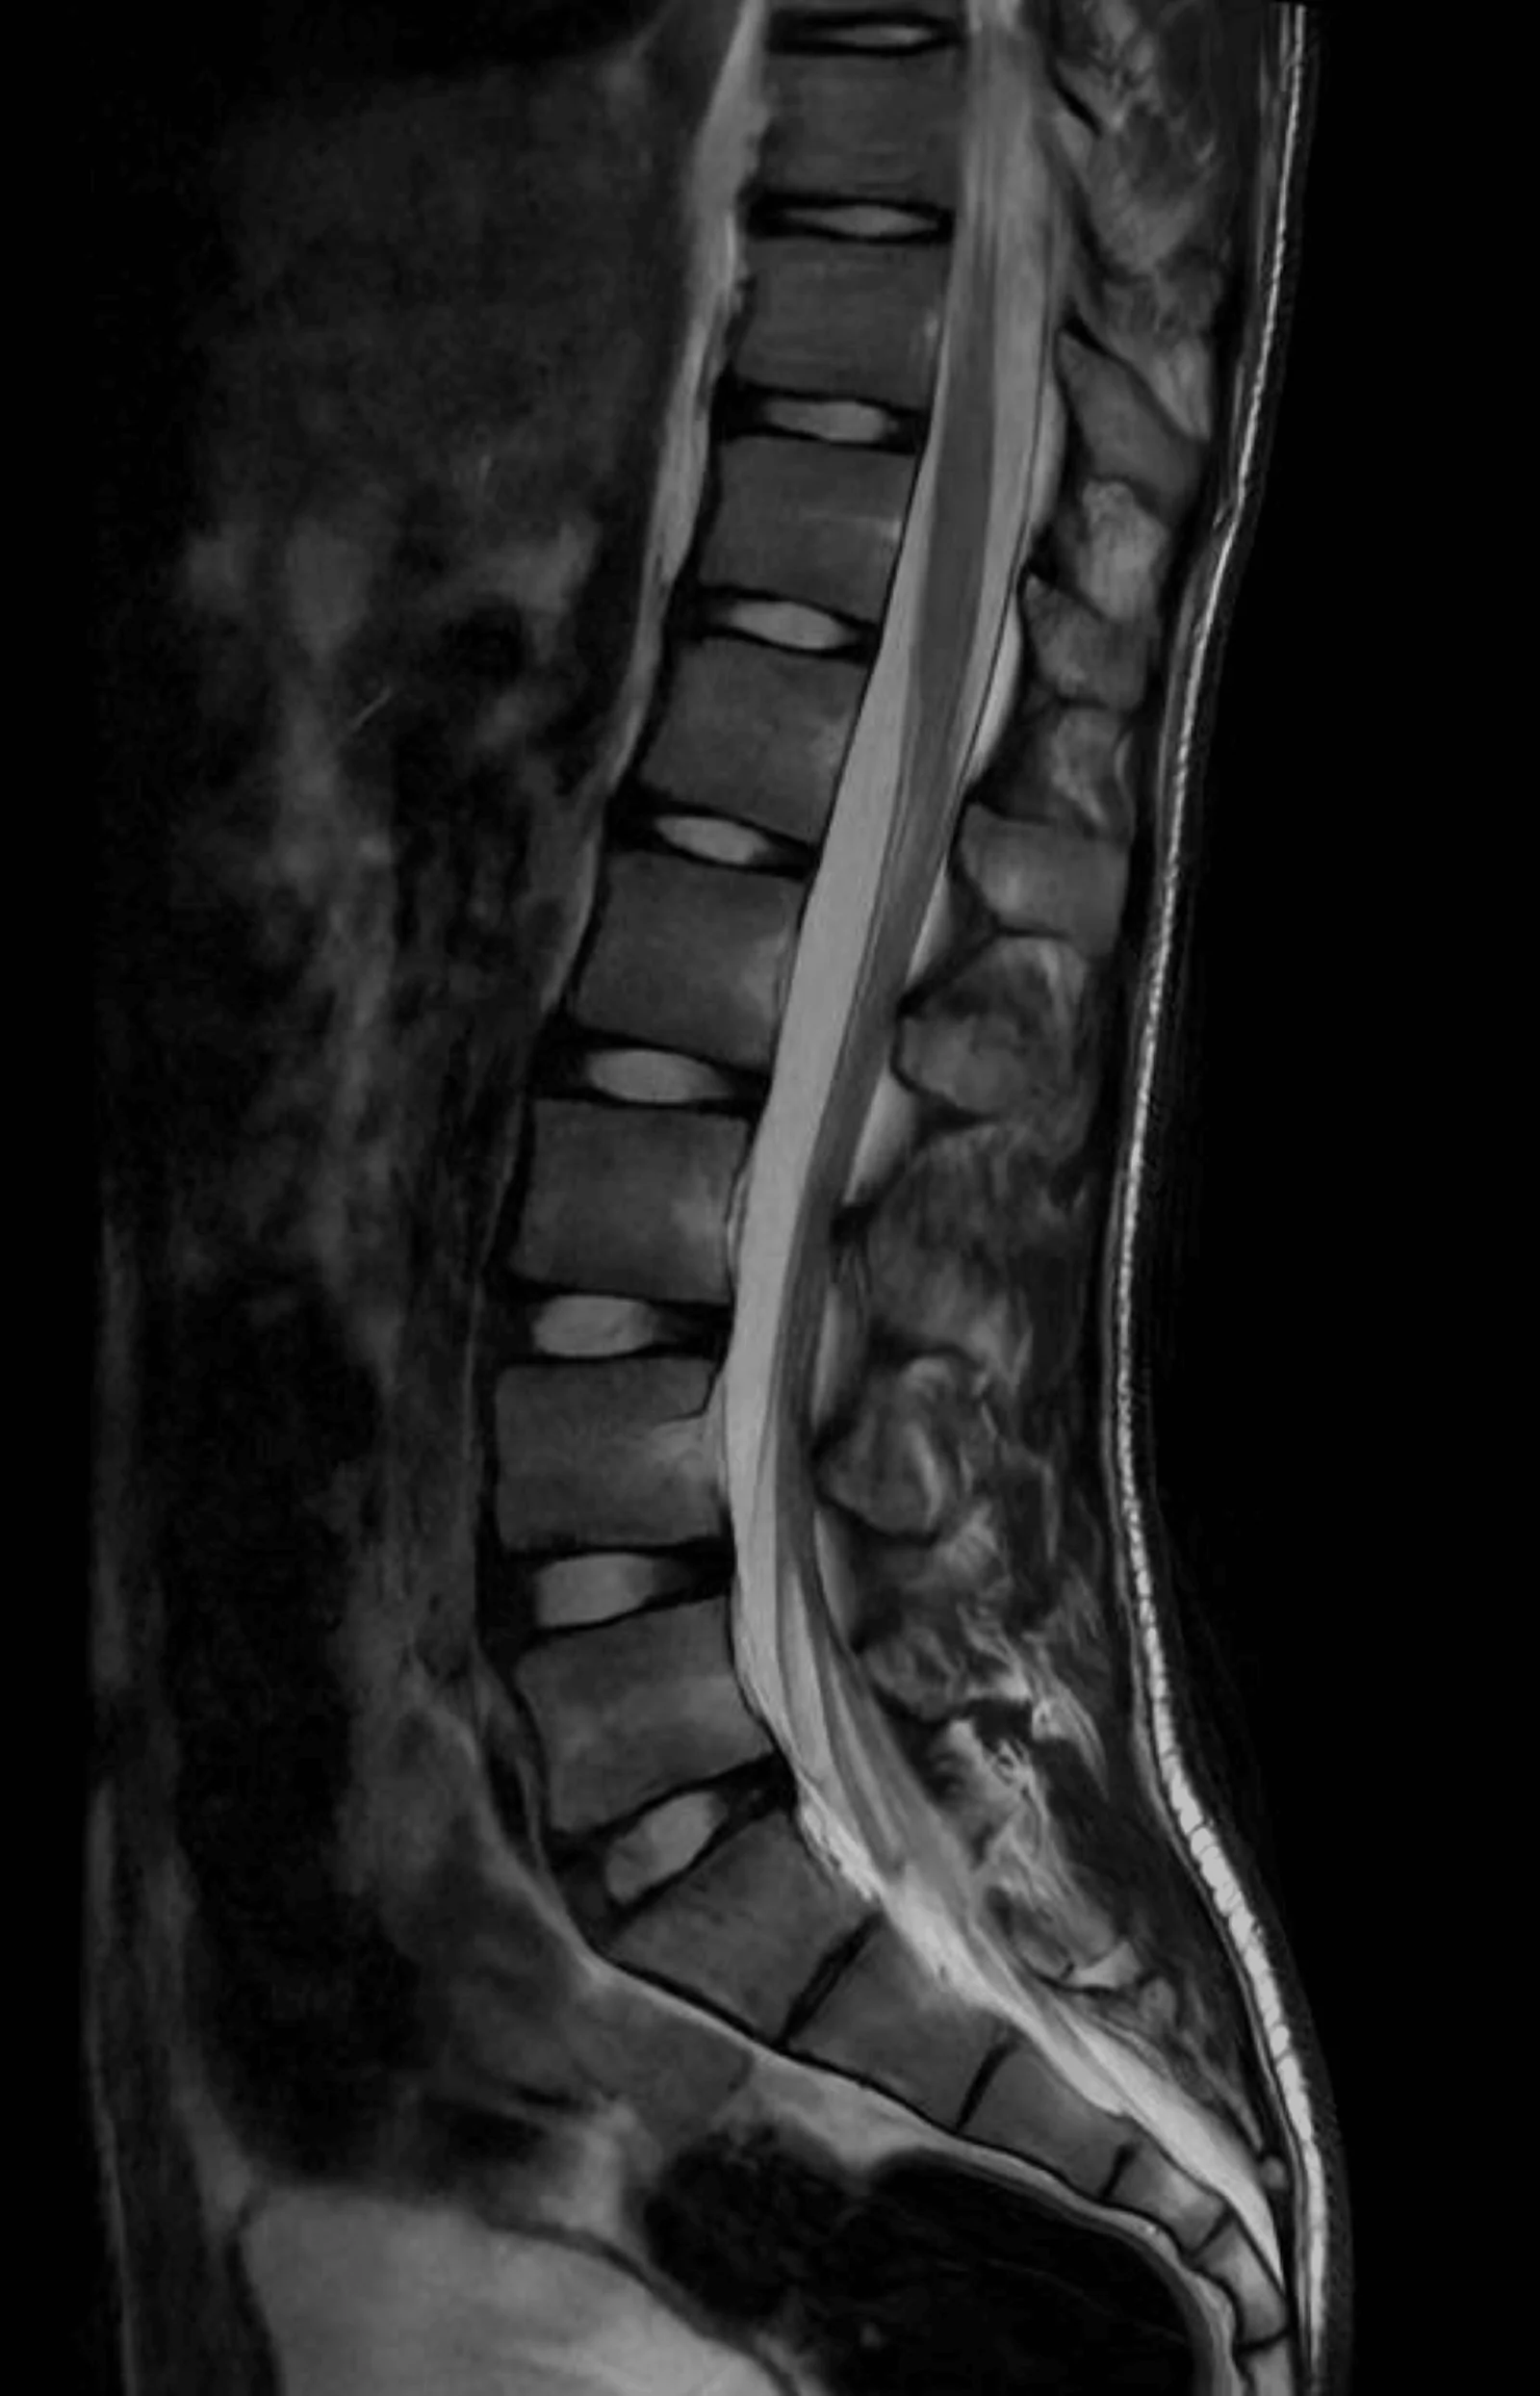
Magnetic resonance imaging (MRI) of the lumbosacral spine demonstrating normal spinal alignment and bone marrow signal with no evidence of discitis or vertebral osteomyelitis.
